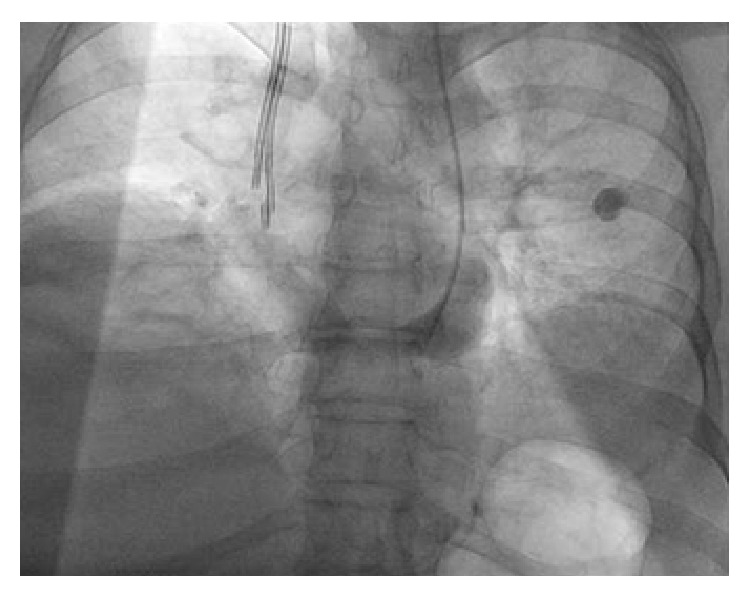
Persistence of left superior vena cava during X-ray fluoroscopic placement of tunnel dialysis catheter.
